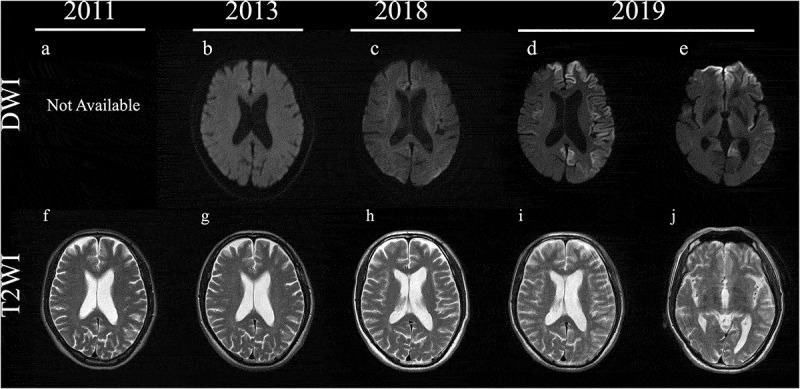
Magnetic resonance imaging (MRI) findings in diffusion-weighted images (DWI) and T2-weighted images (T2WI) in the brain from 2011 to 2019. MRI examinations were normal in DWI and T2WI from 2011 to 2018 (a–c, g–h). In 2019, right dominant cortical high-intensity signals, except in the occipital lobe, in DWI (d) and abnormal swelling in T2WI (i) were detected. The corpus callosum and caudate were normal in DWI and T2WI (c,j).
